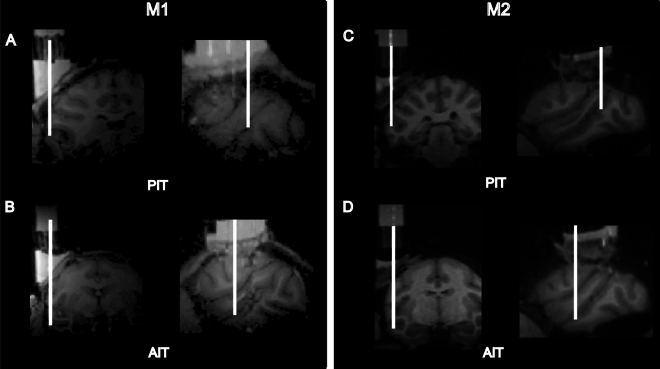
Recording locations in IT. Coronal and sagittal MRI slices of PIT and AIT of the brain of M1 (A, B) and M2 (C, D). The estimated location of an electrode penetration is indicated by a white line.
